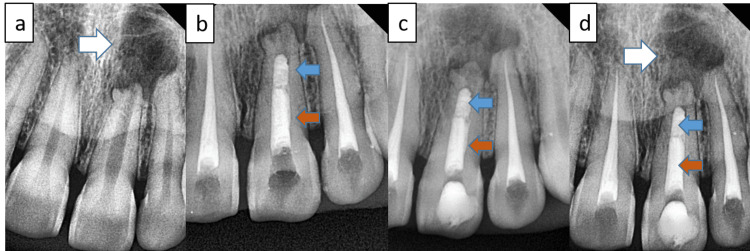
(a) Preoperative radiograph showing the open apex in relation to tooth 21. (b) Radiograph following the modified apexification procedure for tooth 21. (c) Immediate follow-up radiograph. (d) Radiograph taken 12 months post-procedure. The white arrow indicates the preoperative and postoperative radiographs demonstrating a decrease in the size of the periapical lesion. The blue arrow highlights the placement of MTA, while the orange arrow points to the thermoplastic gutta-percha. MTA, mineral trioxide aggregate
